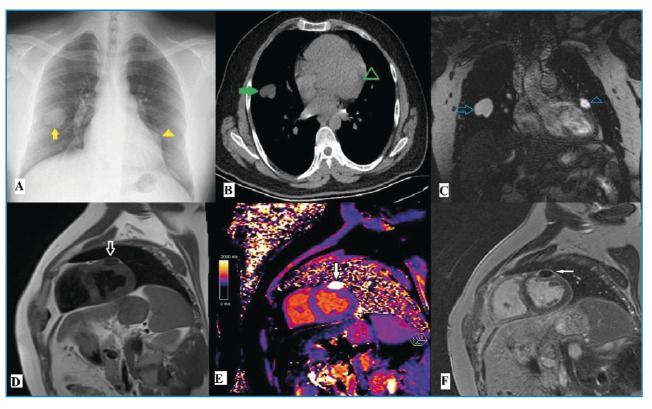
Multimodal imaging of the cardiac hydatid cyst case. A) Chest radiograph showing a radiopaque lesion in the right lung (yellow arrow) and bulging of the left cardiac contour. B) Axial non-contrast computed tomography demonstrating a cystic lesion in the right lung (green arrow) and a hypoattenuating area in the left ventricular wall. C) T2-weighted coronal magnetic resonance (MR) image showing hyperintensity of the lesion in the left ventricular wall (arrowhead). D) T1-weighted short-axis MR image displaying the hypointensity of the same lesion (arrow). E) T1-mapping MR showing prolonged relaxation time. F) Post-contrast cardiac MR demonstrating ring-like enhancement indicative of pericystic fibrotic content.
